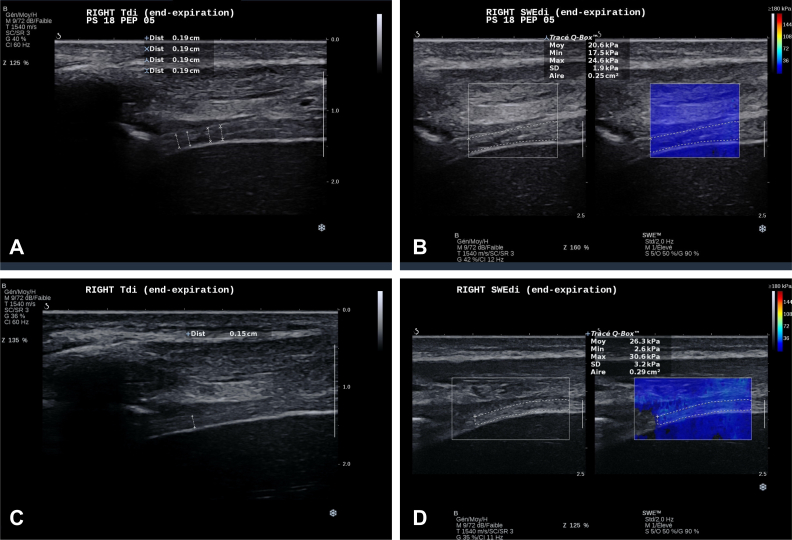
A-D, Right diaphragm at both time points of assessment. A, Right Tdi at end-expiration during high-PS (18 cmH_2_O): first time point of DUS assessment. B, Example right SWEdi at end-expiration during high-PS (18 cmH_2_O): first time point of DUS assessment. C, Right Tdi at end-expiration: second time point of DUS assessment. D, Example right SWEdi at end-expiration: second time point of DUS assessment. Aire = area; Dist = distance; DUS = diaphragm ultrasound scan; Max = maximum; Min = minimum; Moy = mean; PEP = positive expiratory pressure; PS = pressure support; SWEdi = diaphragm shear-wave elastography; Tdi = diaphragm thickness.
